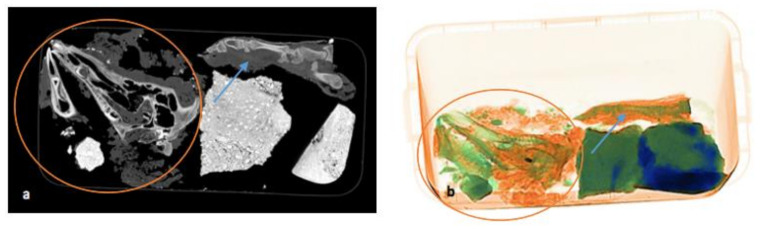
(a,b) Comparison of pmCT (a) and X-ray image (b) on 14 September 2021 during the last scan. The disarticulation of the bones and disintegration of soft tissue (red circle) of the head and intactness of the paw (blue arrow) can be seen.
